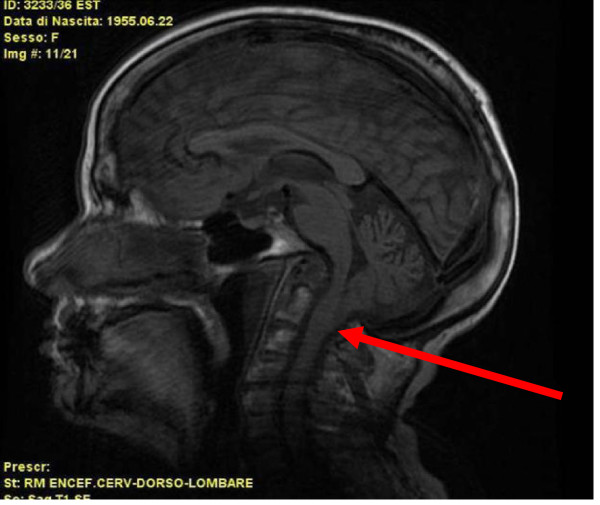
Sagittal craniocervical magnetic resonance imaging section. The arrow shows cerebellar tonsils herniation trough the foramen magnum until the second cervical vertebra and bulbar compression.
